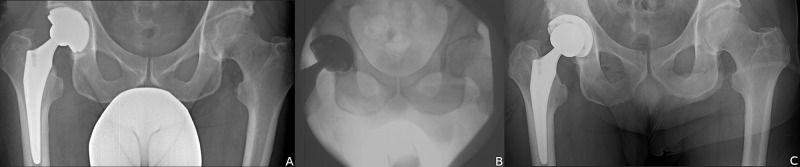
Management of IPI with cup repositioning. (A) Preoperative AP radiograph with vertical and retroverted cup. (B) Intraoperative imaging to confirm cup inclination and version. (C) Follow-up radiograph with well-fixed components and improved cup orientation. IPI, iliopsoas impingement; AP, anteroposterior
